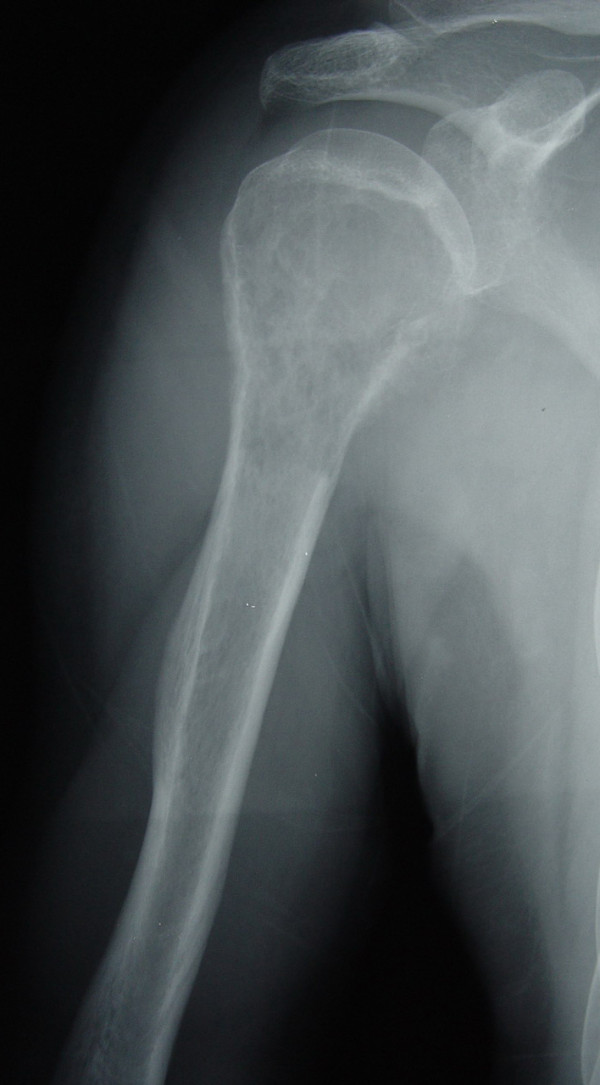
Preoperative X-ray (AP view) of a 35-year male patient with right humeral malignant fibrous histiocytoma.
